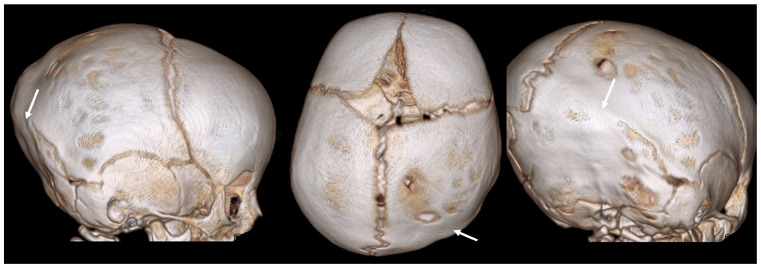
A 3D CT image of a lambdoid CRS (posterior plagiocephaly) with asymmetrical flattening of the back of the head, misalignment of the jaws, ears, and facial structures, and a tilted cranial base. The white arrow indicates an abnormally fused suture.
